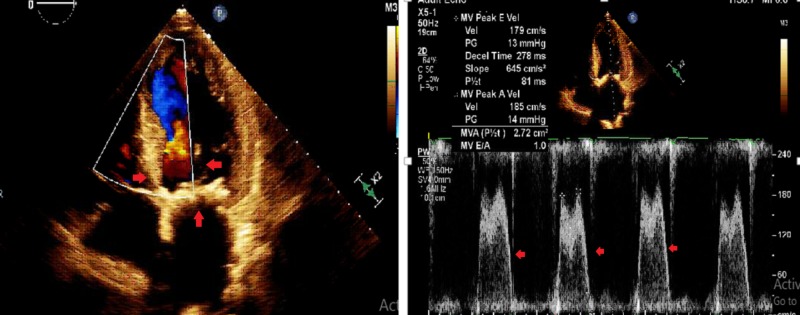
Transthoracic echocardiogram with severe mitral stenosis and elevated gradients across the valve
